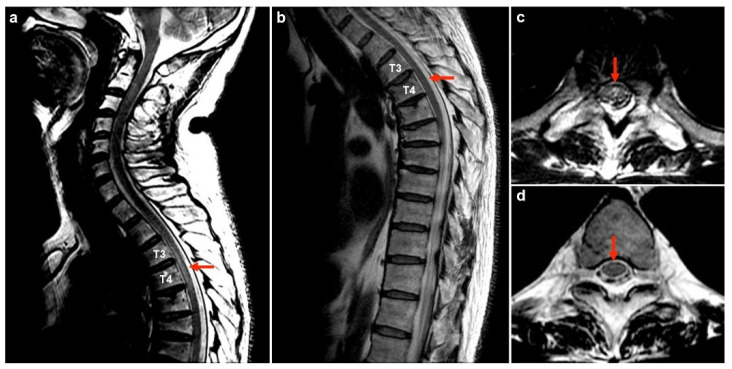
Spinal MRI: On the 6th day after onset, an intramedullary spotty lesion with T2 hyperintensity at T3 to T4 was shown (red arrows: (a) sagittal view. (c) axial view). Five months later, the lesion over T3 to T4 was totally resolved on T2 FLAIR images (red arrows: (b) sagittal view. (d) axial view).
